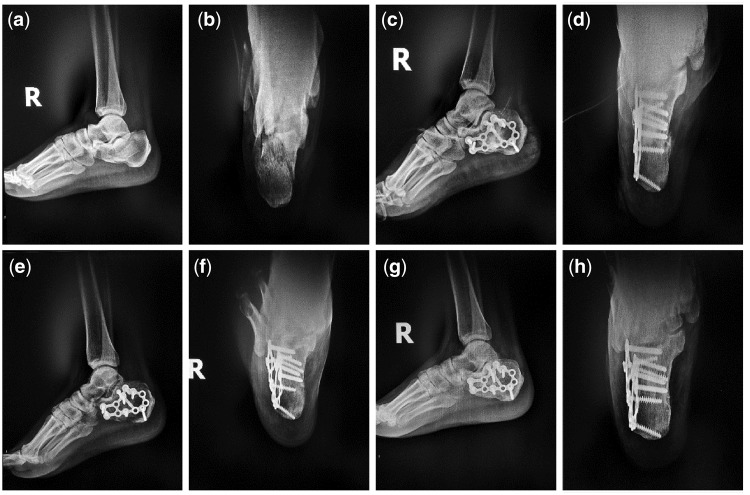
Plain radiographs of the first typical case. (a and b) Preoperative lateral and anteroposterior films; (c and d) immediately postoperative lateral and anteroposterior films; (e and f) 3 months postoperative lateral and anteroposterior films; (g and h) 12 months postoperative lateral and anteroposterior films)
